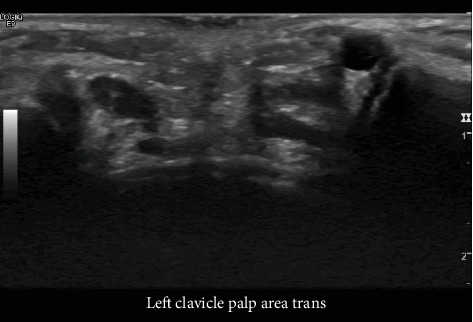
Single sonographic image of the right and left sternoclavicular joints shows a circumscribed, round, cystic mass with increased through transmission located just anterior to the left sternoclavicular joint.
